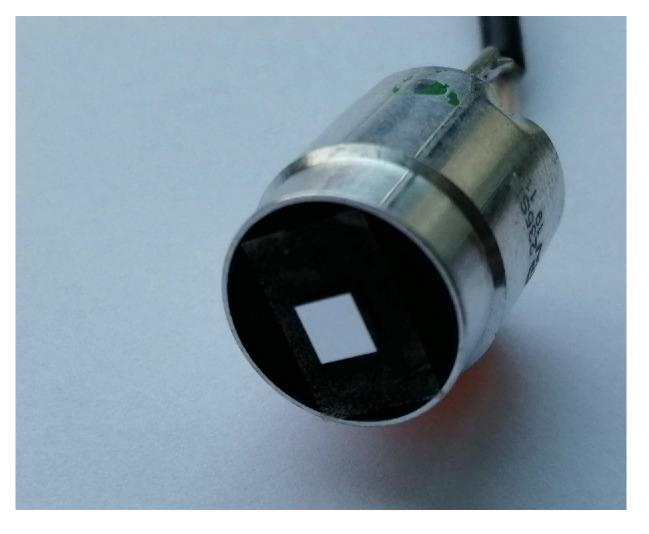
UltraSonic Transducer (*UST*) with measuring mirror for the *SWI*. With this setup, the mirror position can be modulated with a frequency $f_{\mathrm{ca}}$, additionally to the underlying measurement motion.
